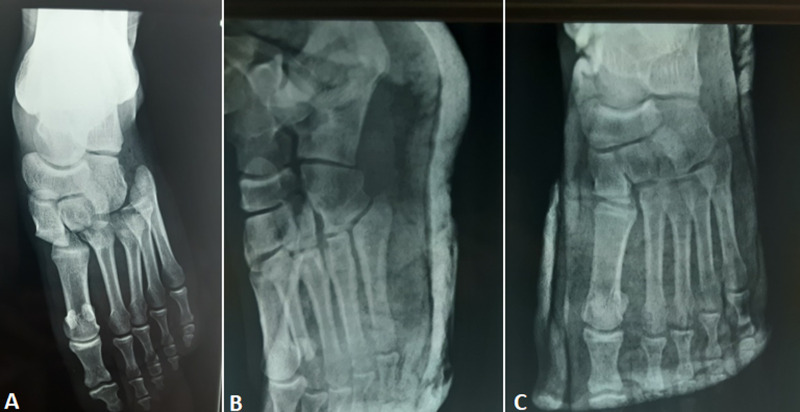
A) foot X-ray with Lisfranc dislocation; B, C) radiological control after reduction
